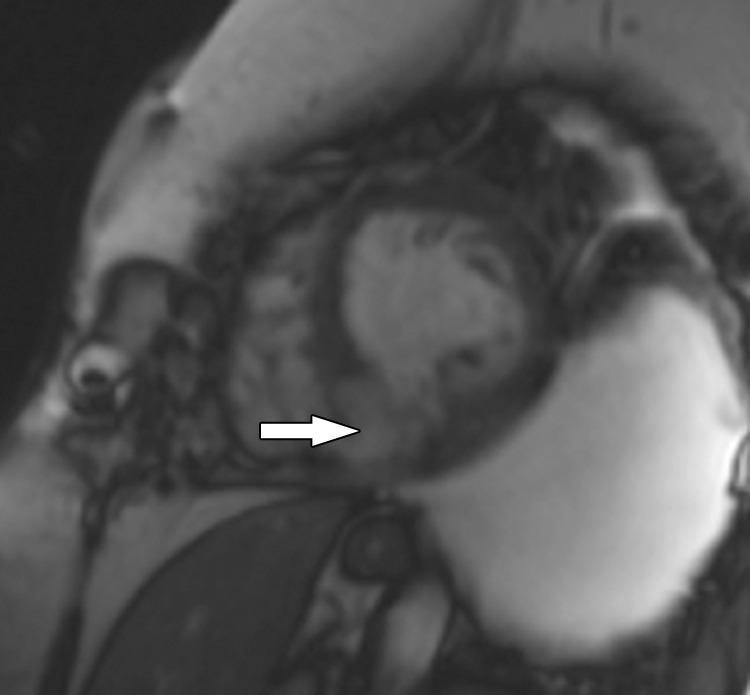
Inferior MI in a 61-year-old woman who developed shortness of breath. Echocardiogram (not shown) depicted an ischemic ventricular septal defect in the location of a prior MI, and the patient was referred for cardiac MRI for further evaluation. Short-axis image from SSFP cine MRI shows an ischemic ventricular septal defect (arrow) between the left and right ventricles at the site of the MI. Supplemental MRI cine clips of the short axis and left ventricular outflow show a flow jet from the LV into the right ventricle through the ischemic ventricular septal defect (Movies 1, 2).
